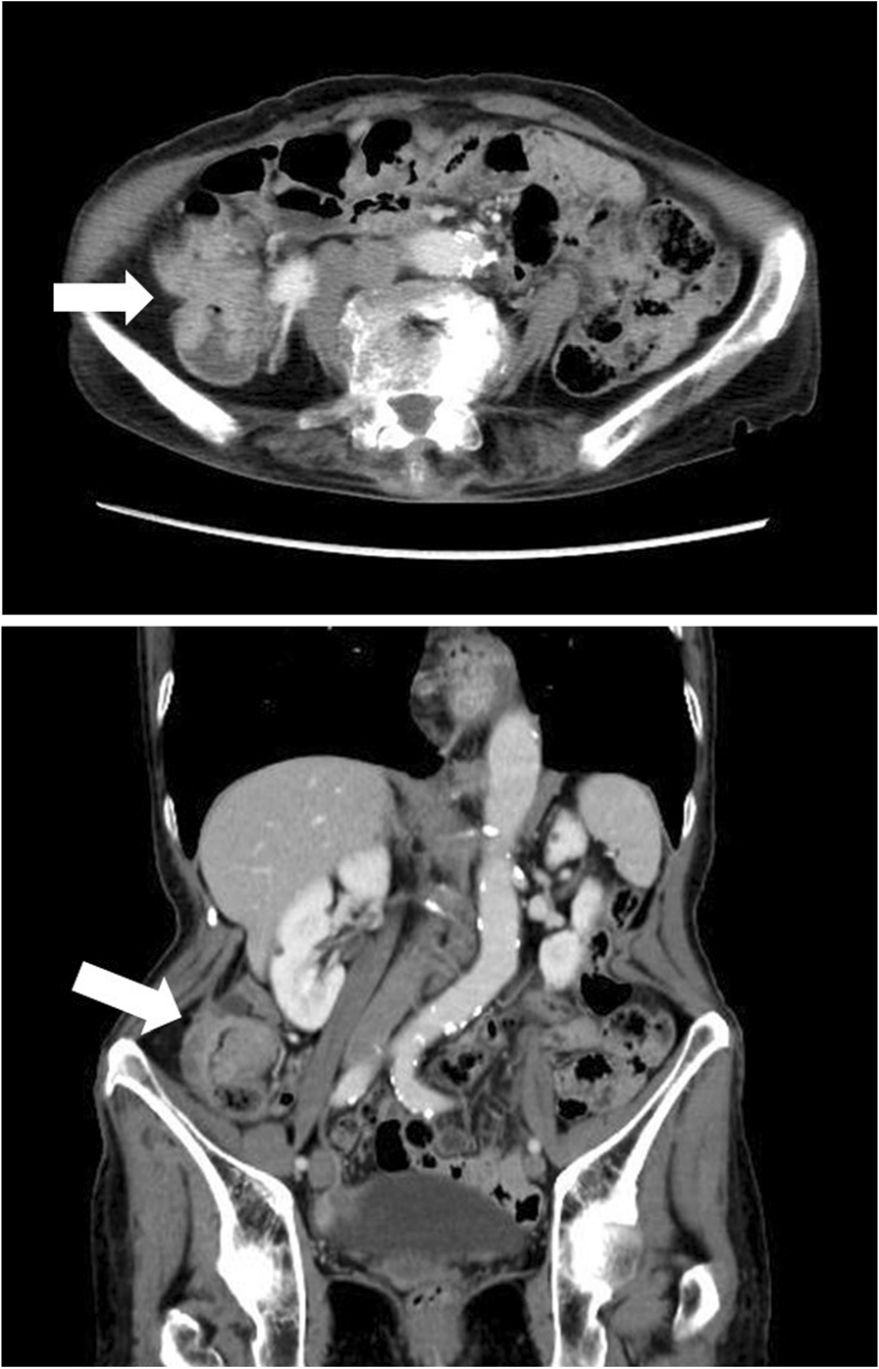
CT: the wall thickness of the ascending colon was observed
